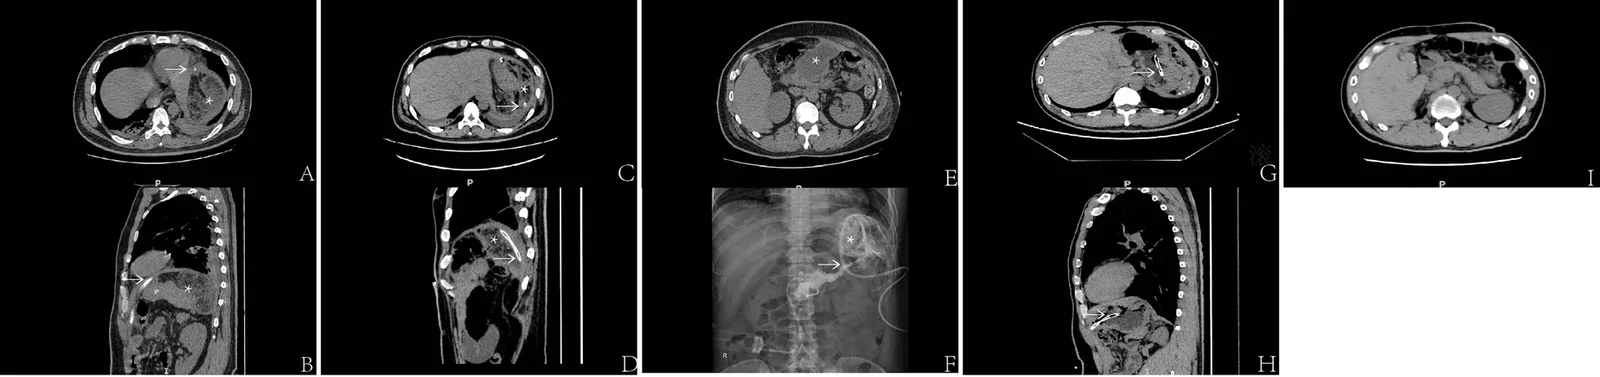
Imaging findings during the clinical course. (A,B) Initial CT after transfer showing fluid collection in the splenectomy bed and an encapsulated collection anterior to the pancreatic body; the original drain was not located within the collection. (C,D) CT after placement of an 18-Fr silicone tube through the original sinus tract. (E,F) Drain tract contrast study showing communication between the peripancreatic drain and the splenic fossa drain. (G,H) CT at readmission showing resolution of the previous collections, with the splenic fossa drain visible within the stomach. (I) Follow-up CT three days after complete drain removal showing no recurrent intra-abdominal collection. Arrows indicate drainage tubes, and asterisks indicate abscess cavities or encapsulated collections.
